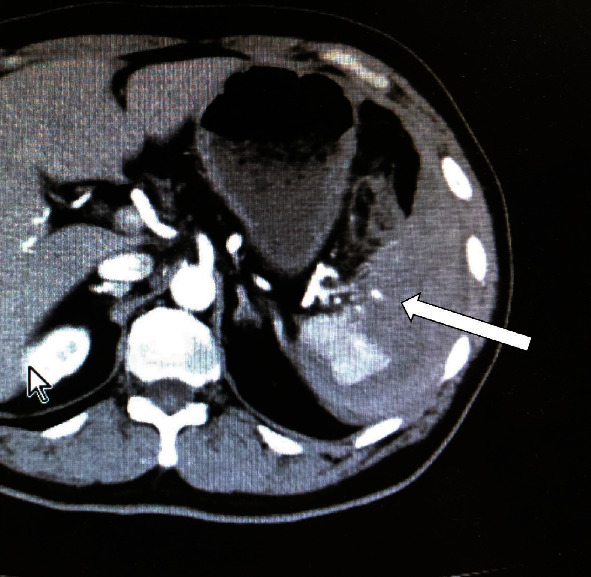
The contrast-enhanced computed tomography (CT) scan of the abdomen showed a shattered spleen and contrast blush (arrow).
